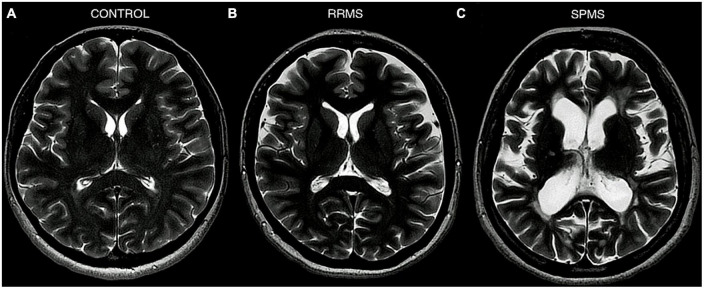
MRI T2-weighted sequence, axial section. Comparison of the degree of brain atrophy and brain ventricular volume between healthy controls (A), patients with RRMS (B), and patients with SPMS (C). Material from the resources of the Medical Radiology Department of the Military Medical Institute–National Research Institute.
